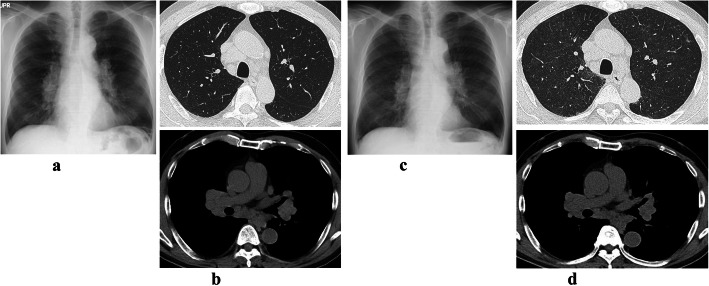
Chest X-ray and CT at diagnosis of sarcoidosis complicated by acute hypersensitivity pneumonitis. Past medical history included sarcoidosis with bilateral hilar and mediastinal lymphadenopathy on chest X-ray (a) and CT (b) and elevated level of serum angiotensin-converting enzyme and lysozyme 4 years earlier. Chest X-ray (c) and CT (d) just before admission in March 2017 showing new bilateral diffuse ground-glass opacities with partial centrilobular distribution
